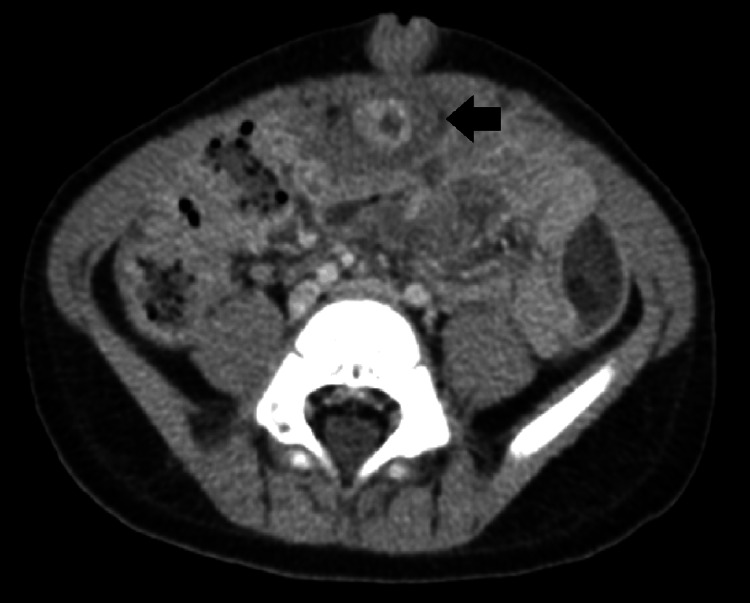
Computed tomography (CT) CT demonstrated an infraumbilical abscess (black arrow).
